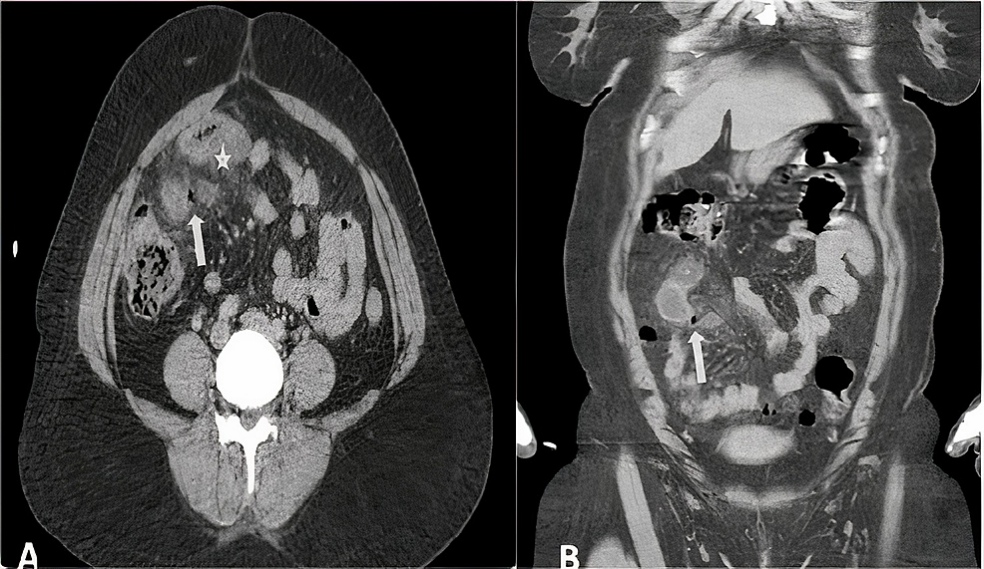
Abdominal computed tomography image. Edema in the small bowel (asterisk) and millimetric air density (arrows).
